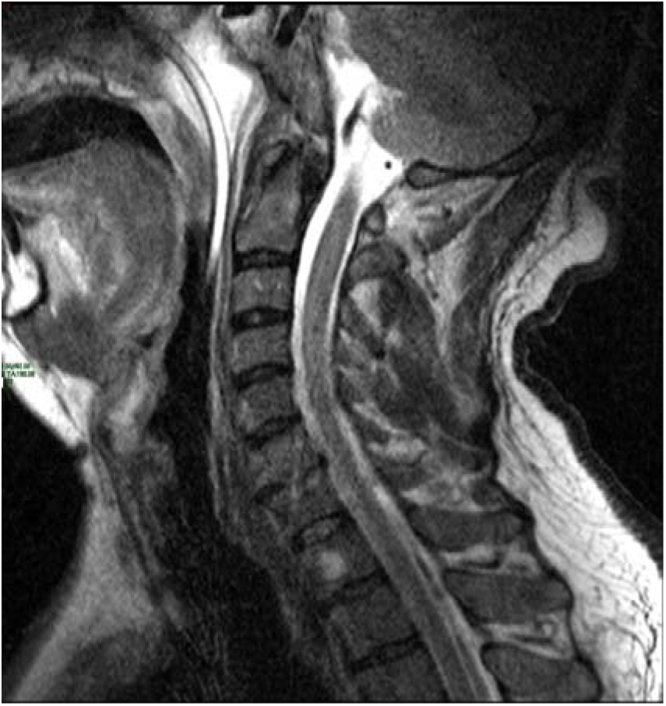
MRI cervical spine revealed no abnormalities in the spinal cord.
